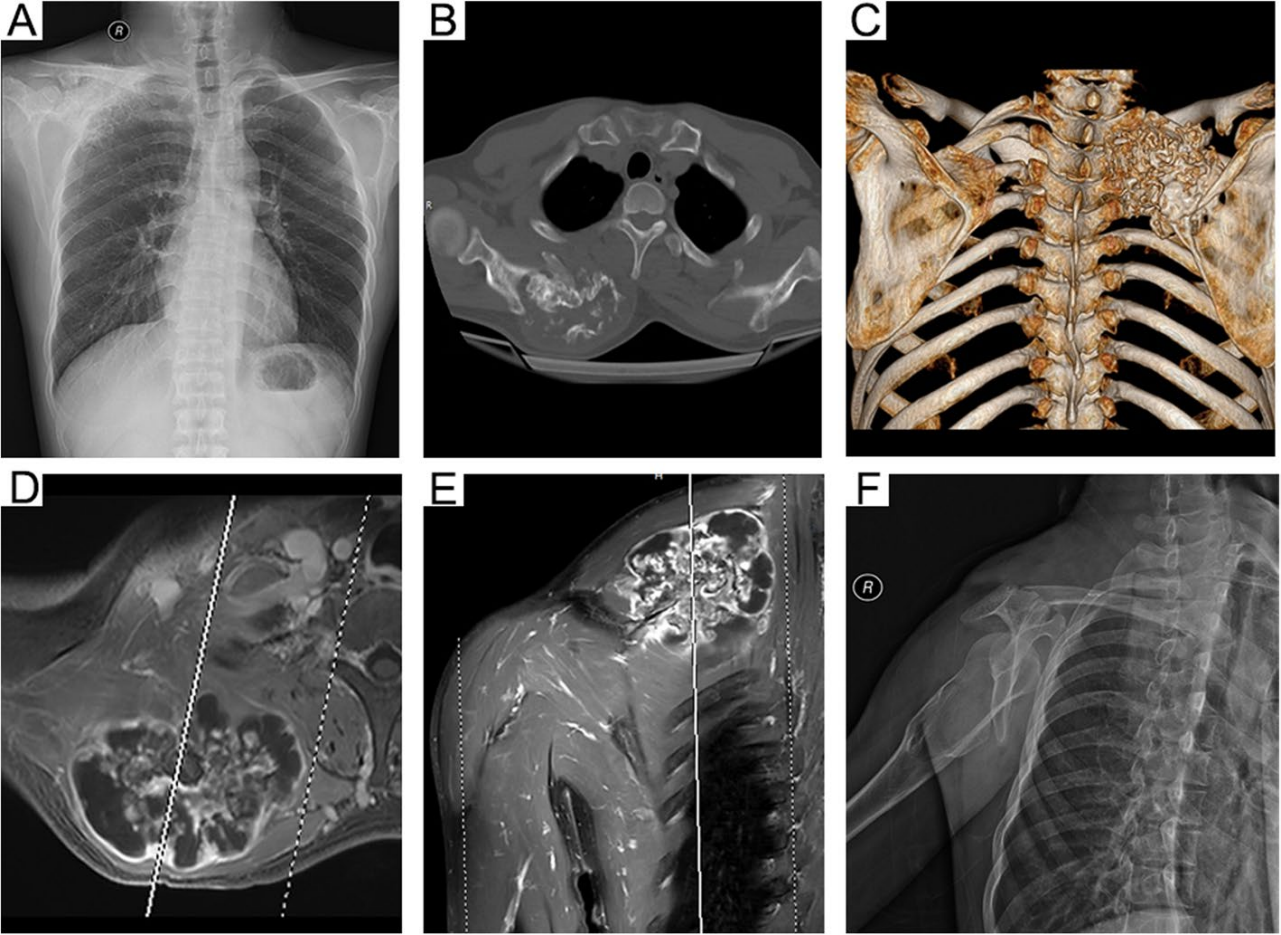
A 27-year-old male with right scapular common chondrosarcoma due to malignant transformation of hereditary multiple osteochondromas (case #6 in Table 1). A: Preoperative anteroposterior plain radiograph. B-C: Cross-sectional CT scan and three-dimensional reconstruction imaging. D-E: Magnetic resonance imaging (T1WI cross-section and coronal). F: Postoperative anteroposterior X-radiograph imaging
